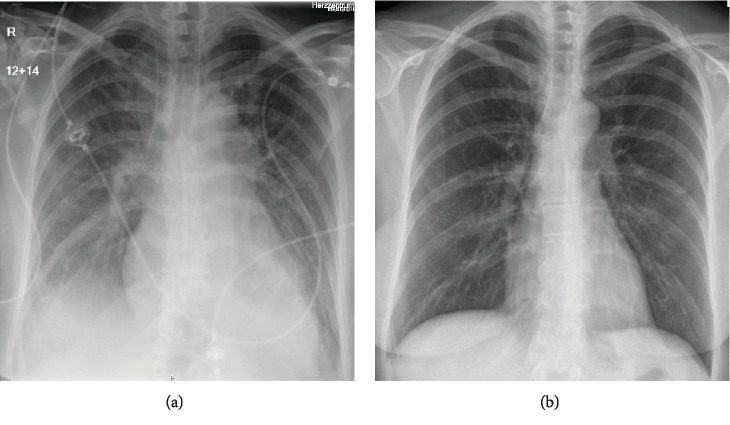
Chest X-ray on admission (a) showing signs of heart enlargement and pleural effusion. Six days later before discharge (b), the heart size is normal again, and there is complete remission of pleural effusion.
